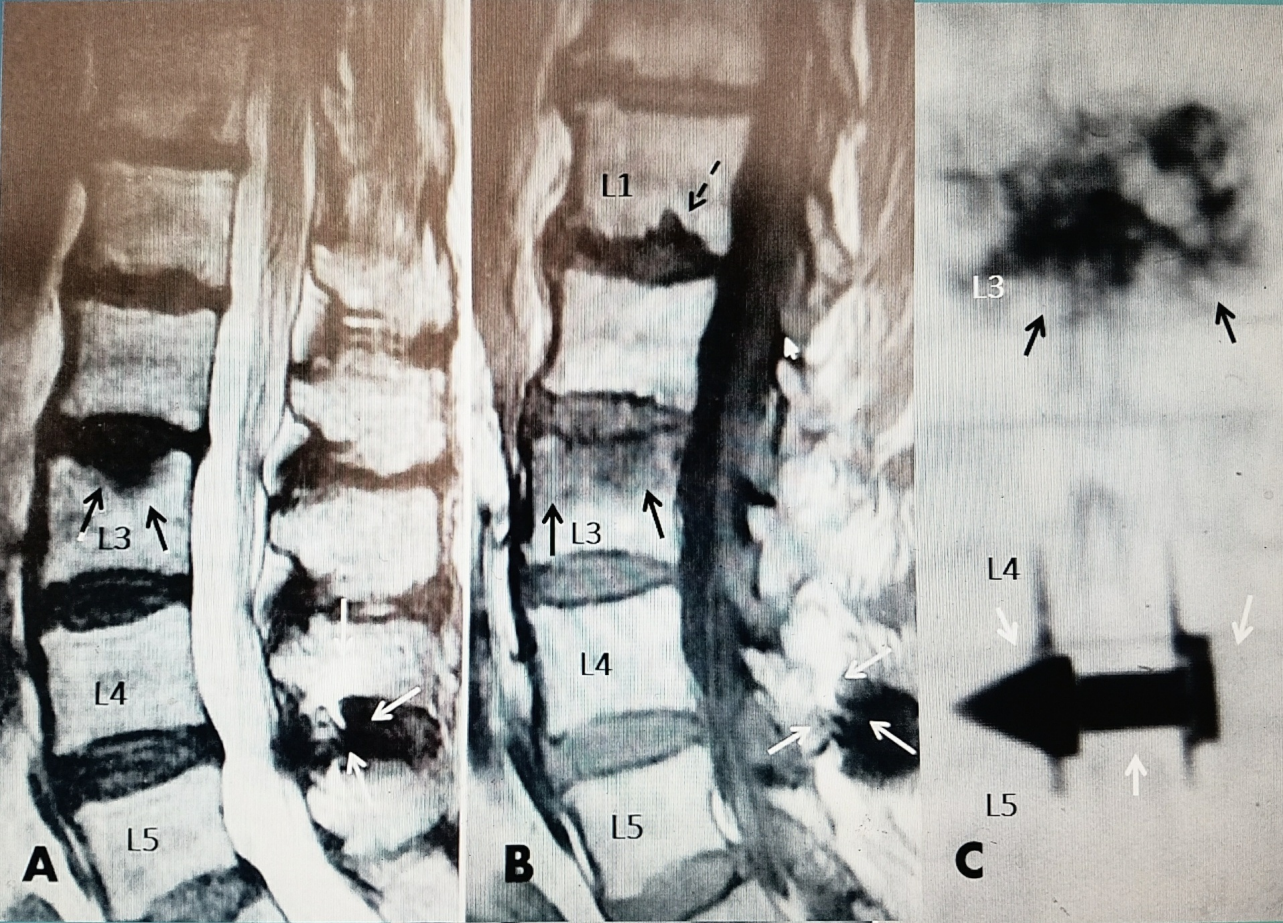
L3 osteoporotic compression fracture five years after L4-5 interspinous distraction device A: Sagittal T2 (MRI) showing acute L3 superior endplate compression fracture (solid black arrows) above L4-5 interspinous distraction device (solid white arrows). B: T1 sagittal MRI showing 50% edema in superior L3 vertebrae (solid black arrows). There is a Schmorl's node or possible second fracture at the inferior endplate of L1 (dotted black arrow). Interspinous device solid white arrows at L4-5. C: Postoperative AP X-rays showing bilateral vertebroplasty cement at L3 (black arrows) and L4-5 interspinous distraction device (white arrows).
